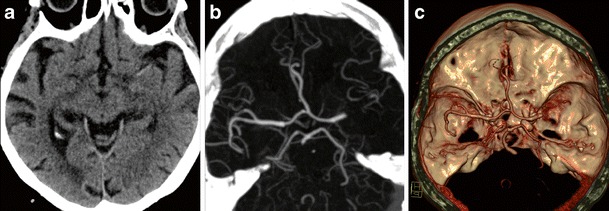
Acute obliteration of the left MCA in an 82-year-old female. a Hyperdense artery sign suggesting an acute thrombus in the left MCA. b CT angiography shows obliteration of the left MCA. c VRT reconstruction. MCA, middle cerebral artery; VRT, volume-rendering technique
